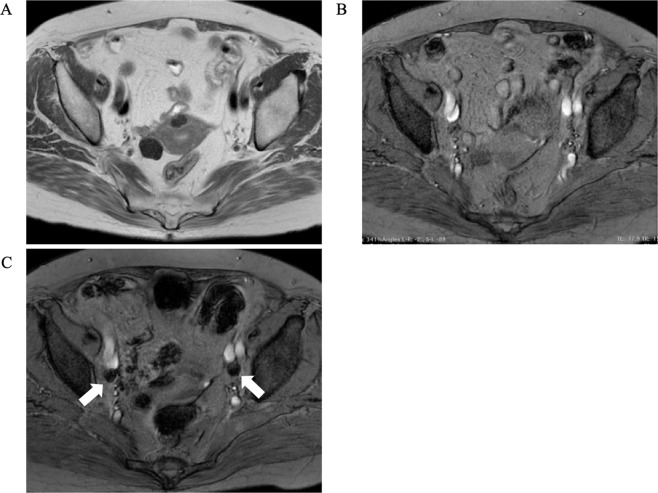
Representative SPIO/MRI images. (A) Pre-SPIO MRI T2-weighted image (axial); (B) pre-SPIO MRI T2*-weighted image (axial); (C) post-SPIO MRI T2*-weighted image (axial). LNs that have taken up SPIO show as enhanced black (white arrows). LN: lymph node; MRI: magnetic resonance imaging; SPIO: superparamagnetic iron oxide.
